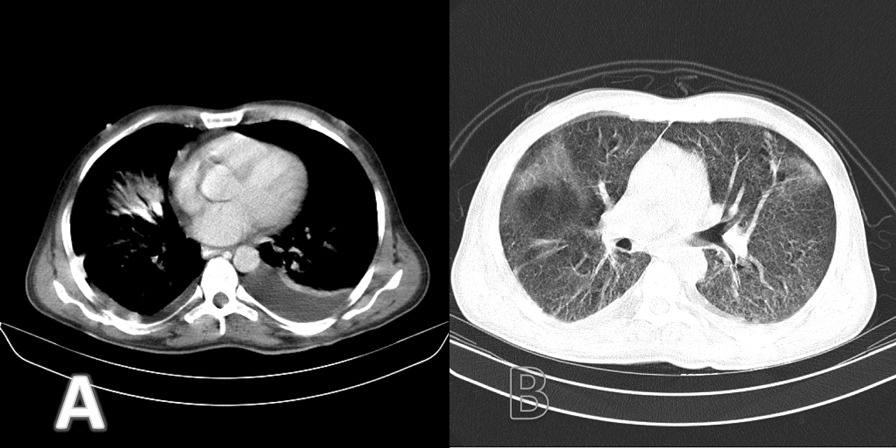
Chest computed tomography with intravenous contrast; A mediastinal window showing left-sided dominant pleural effusion, B parenchymal window showing diffuse ground-glass opacities
